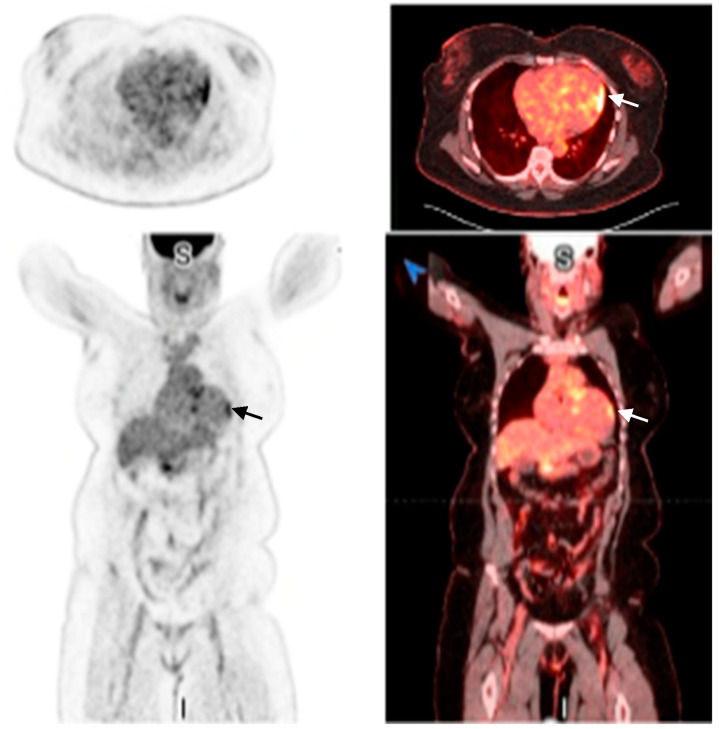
FDG PET-CT showing myocardial FDG uptake (arrows).
